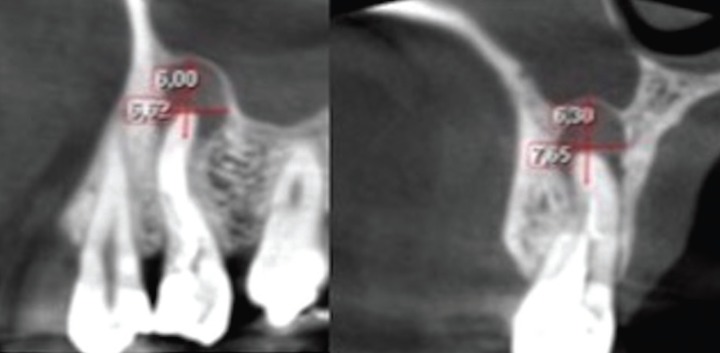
Sagittal (A) and coronal (B) sections used to obtain the measurements of the periapical area of tooth 15.
